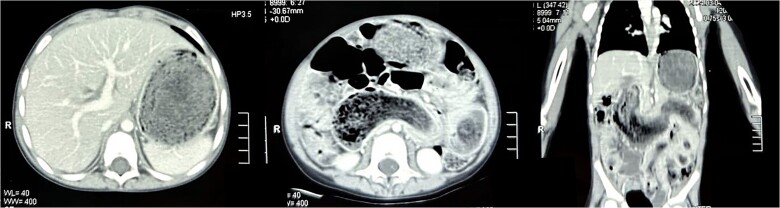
Transverse section of the CT scan of the abdomen revealing non-contrast enhancing, well-circumscribed homogenous mass in the stomach (left). Coronal section of the CT scan of the abdomen revealing the mass extending from the stomach all the way into jejunum (right).
